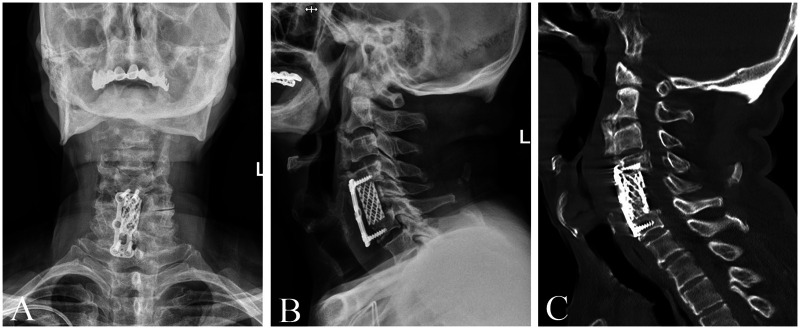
(A,B) cervical spine radiographs (AP and lateral views) at 2-month follow-up. (C) Postoperative follow-up cervical spine CT.
